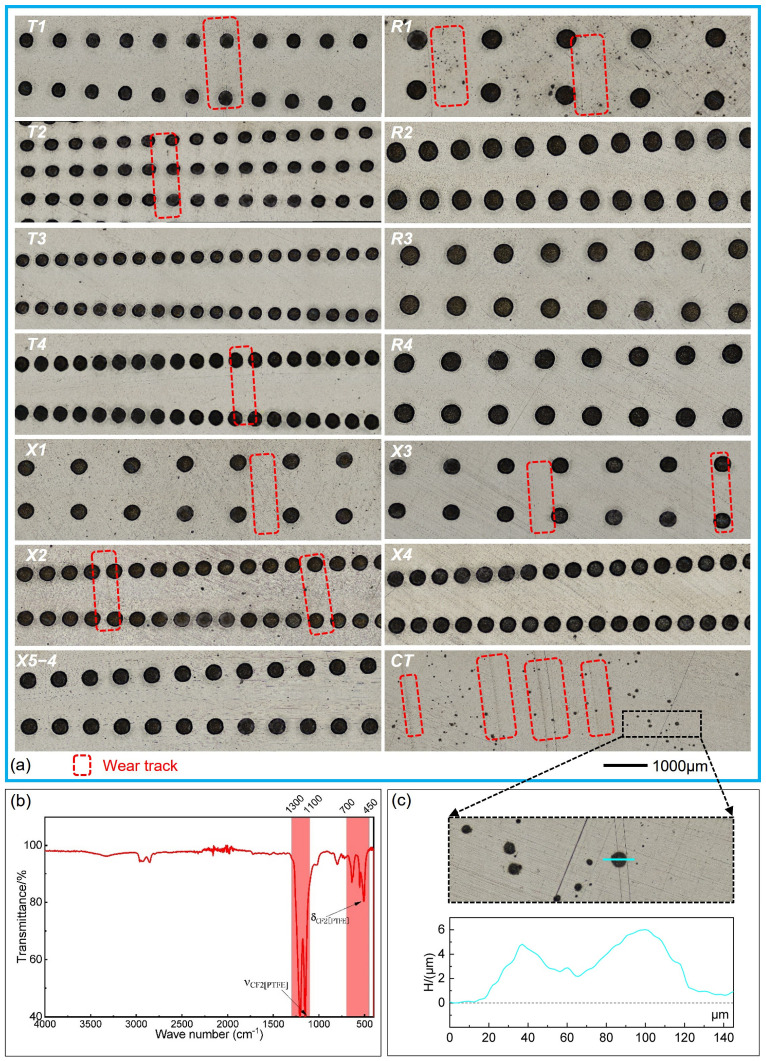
Representative worn surfaces of the 40# steel discs of different groups after wear tests and the FTIR curve of the PTFE transfer film. (a) Worn surfaces of the 40# steel discs after ultrasonic cleaning. (b) Typical infrared spectral characteristics of the PTFE debris collected from the transfer film on the contact surface of the 40# steel disc. (c) Section view of one black spot on the worn surface of the steel disc of the CT group.
